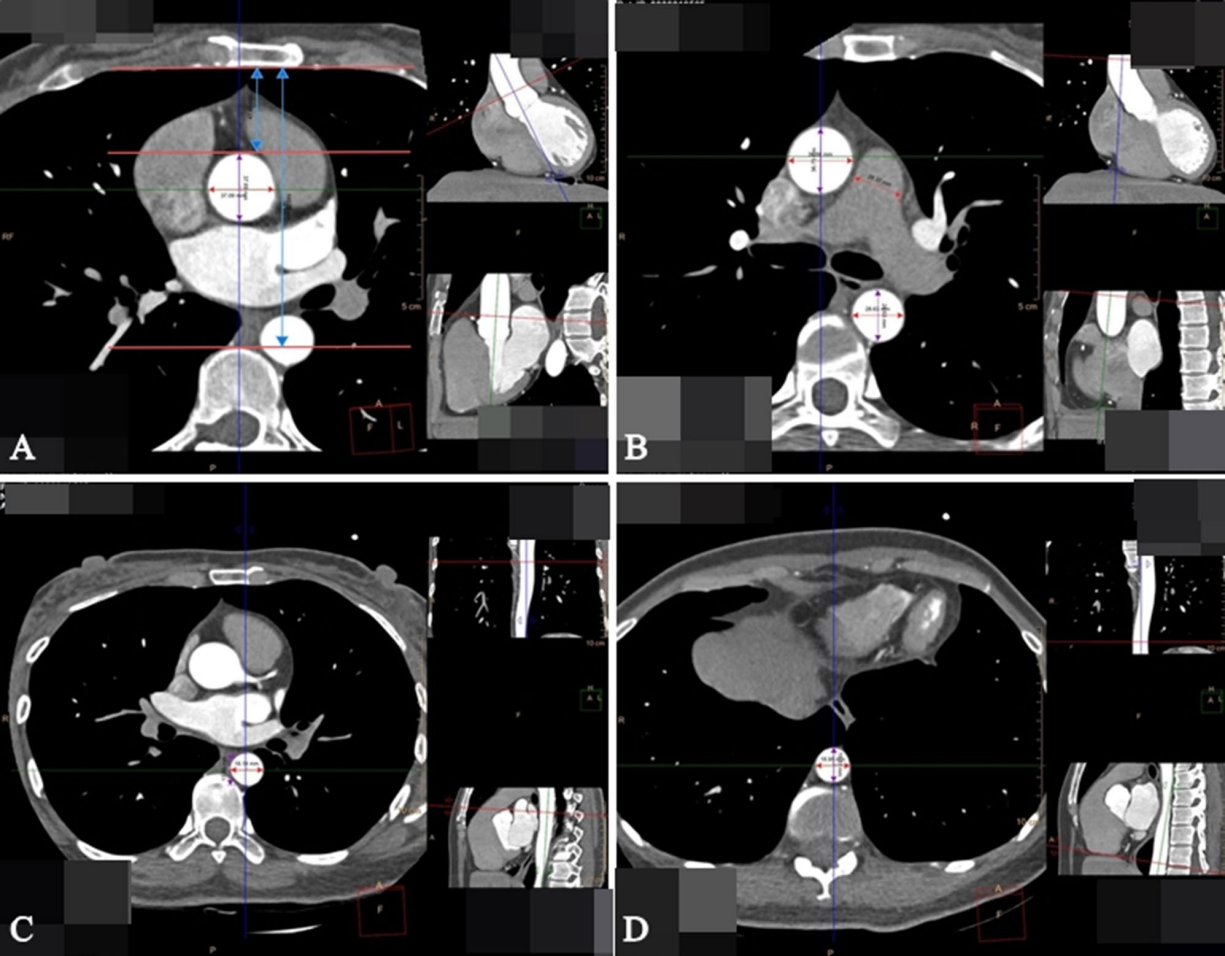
Axial contrast enhanced CT thorax with cardiac gated CT protocol were performed and representative slices were include at the levels of (A) The plane of sinus junction—the transverse and anteroposterior diameter of the ascending aorta, the distance from sternum to ascending aorta and to spinal distances. (B) The plane of pulmonary bifurcation—the transverse and anteroposterior diameter of the ascending aorta, descending aorta, and the diameter of the main pulmonary artery. (C) The plane of sinus junction—the transverse and anteroposterior diameter of descending aorta. (D) The plane of the diaphragm—the transverse and anteroposterior diameter of descending aorta.
